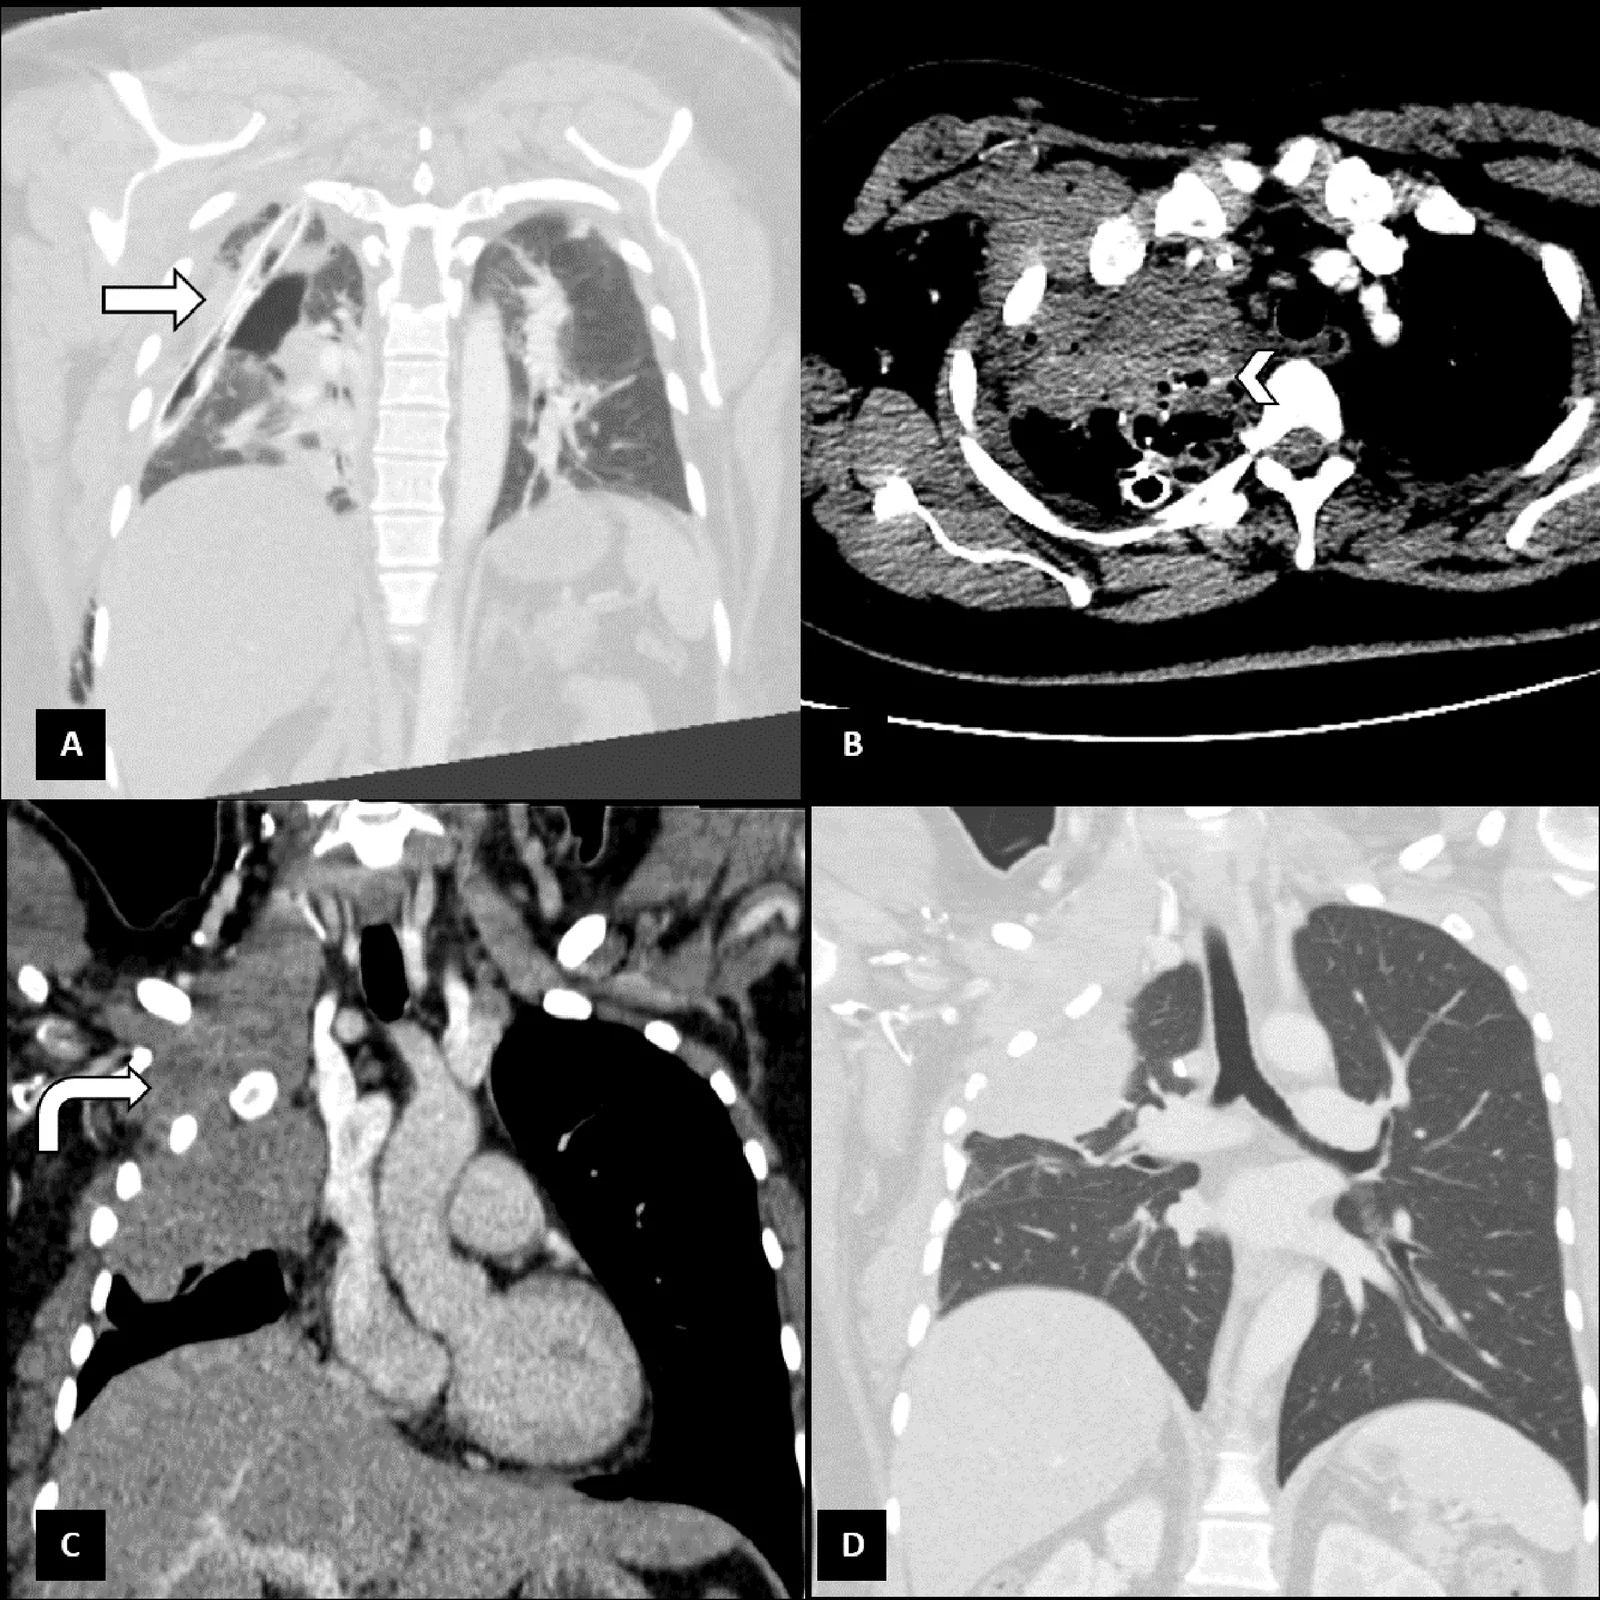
Postoperative and follow-up contrast-enhanced chest CT. (A and B) Images obtained 8 days after surgery. (A) Coronal lung window shows a chest tube and right pneumothorax (arrow). (B) Axial soft-tissue window demonstrates postoperative atelectasis (arrowhead) and residual tumor extending into adjacent soft tissues. (C and D) CT obtained 30 months later shows persistent and progressive residual disease. (C) Coronal image demonstrates involvement of the right superior thoracic inlet with sparing of the contralateral side. (D) Coronal lung window shows mass effect on the adjacent pulmonary parenchyma.
